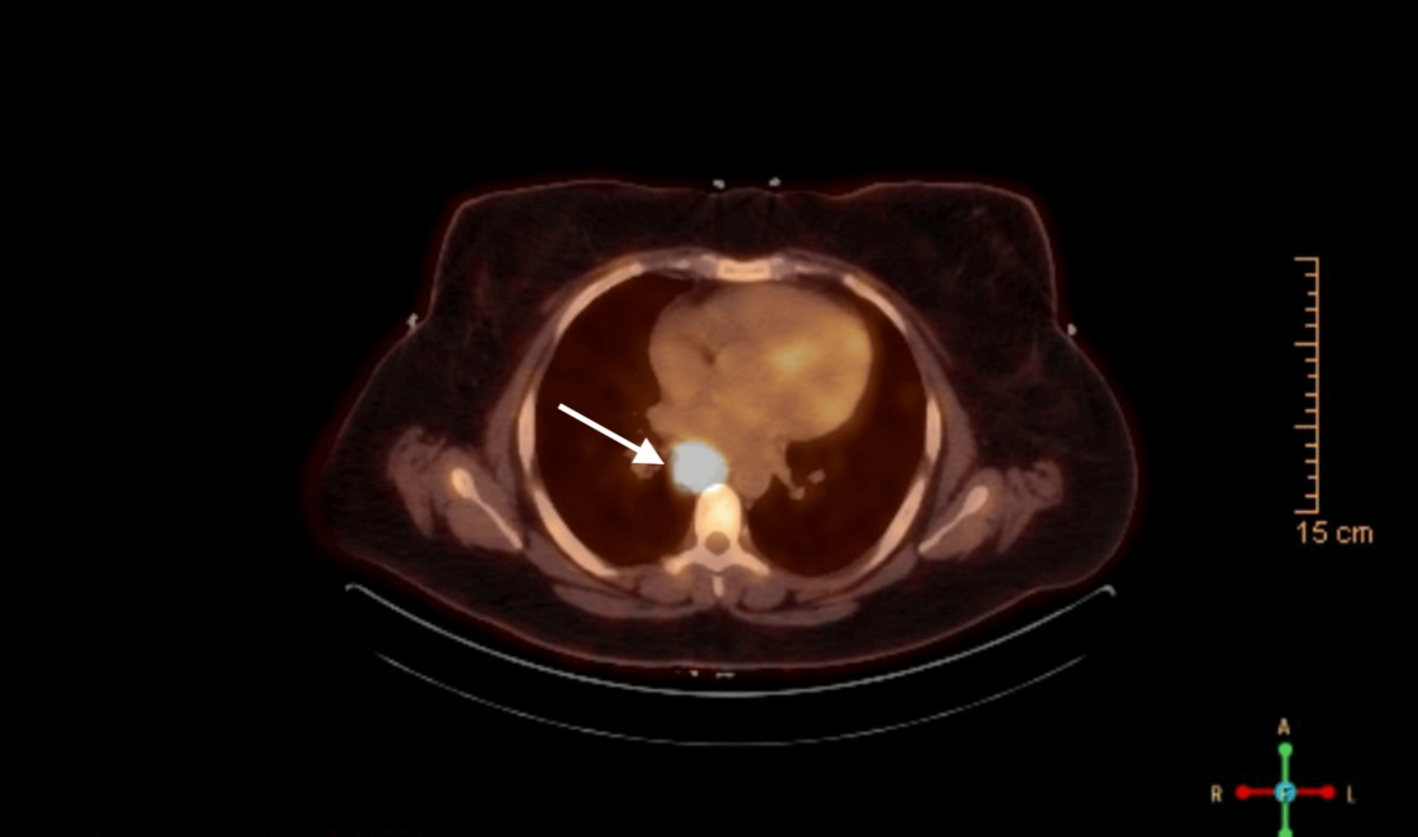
PET-CT scan demonstrating FDG-avid subcarinal lymph nodes PET-CT: positron emission tomography-computed tomography; FDG-avid: fluorodeoxyglucose-avid
